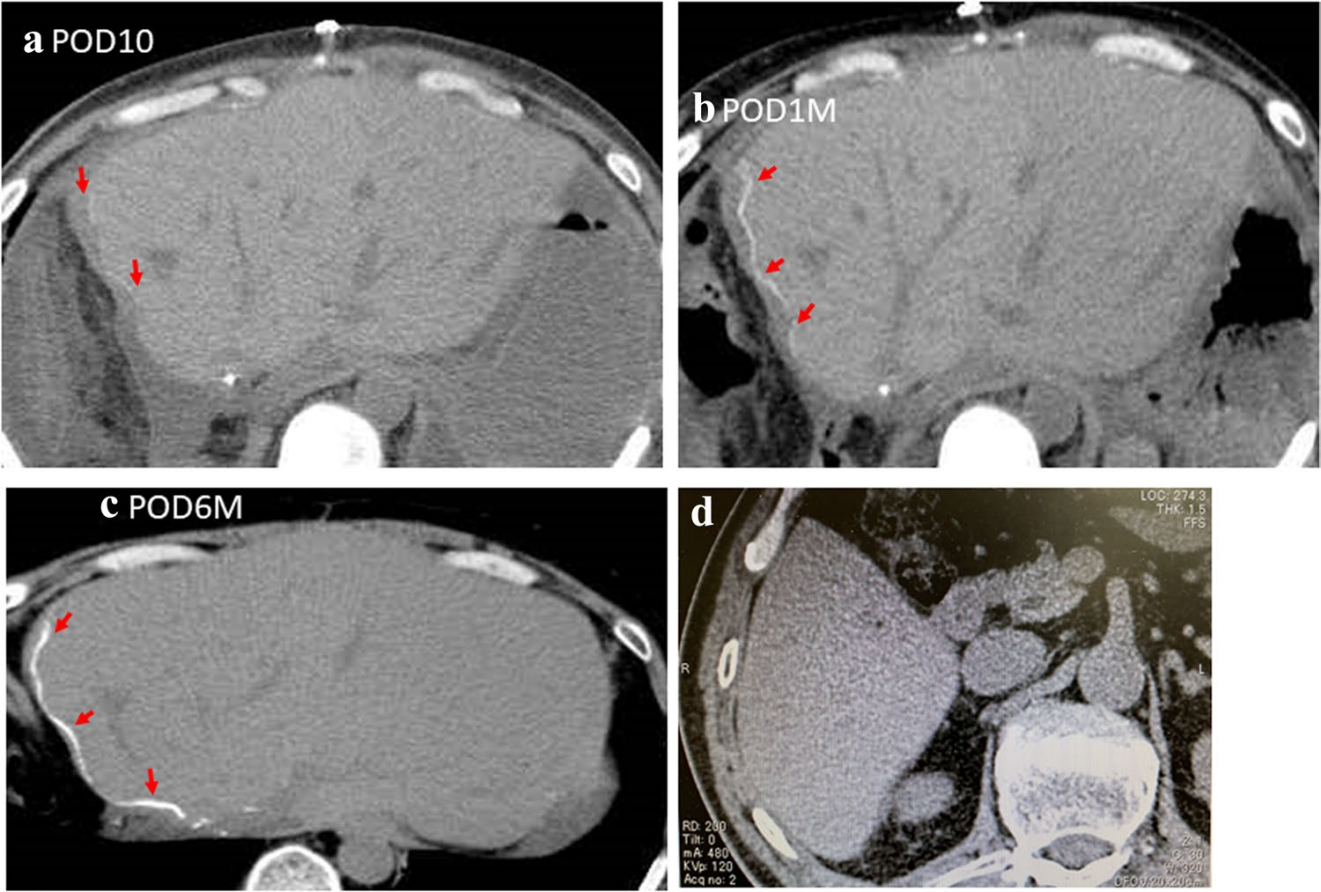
CT image from case 1 showing the accumulation of calcium in the surrounding tissues of the wound. a POD10, the surrounding tissue of the wound showed slight calcification. b One month after LDLT, the calcification had increased. c Six months after LDLT, the graft calcification had further increased. d Postoperative CT image showed on presence of calcification in the donor’s liver
